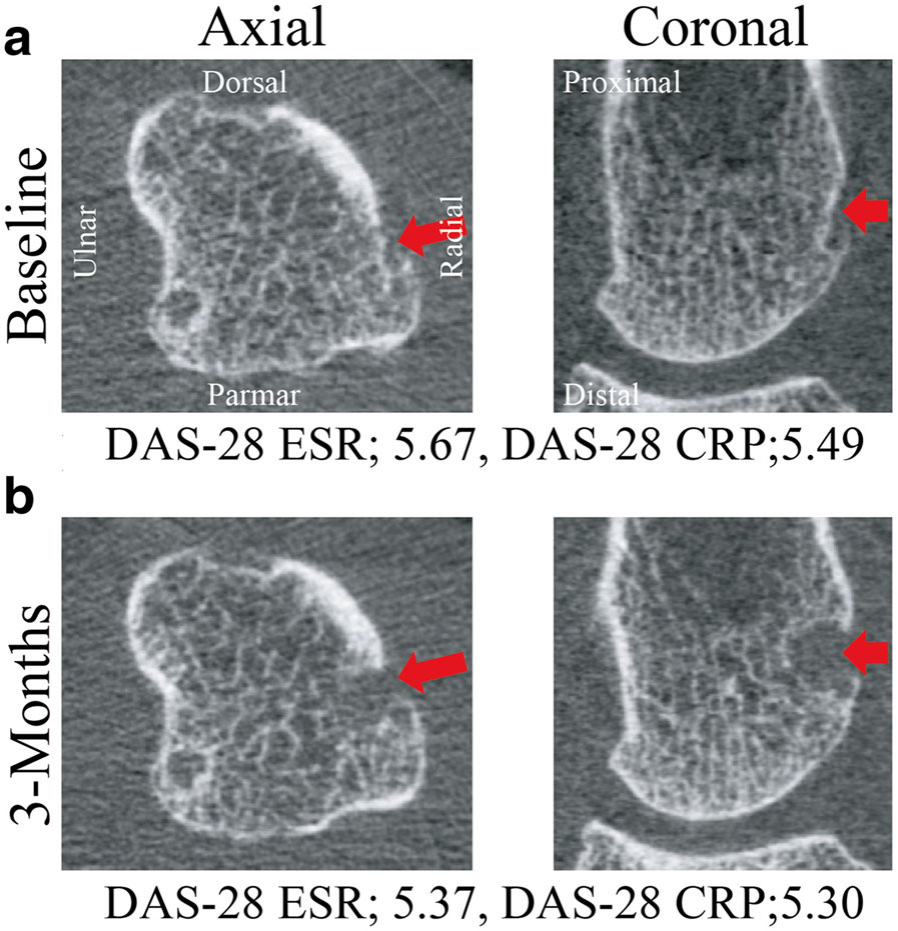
Axial and coronal high-resolution peripheral quantitative computed tomography (HR-pQCT) images at the second metacarpal head in a patient who demonstrated increased erosion (erosion volume = 3.90 mm^3^ at baseline (a) and 12.38 mm^3^ at 3 months (b)). Red arrows indicate bone erosion. DAS-28 disease activity score in 28 joints
